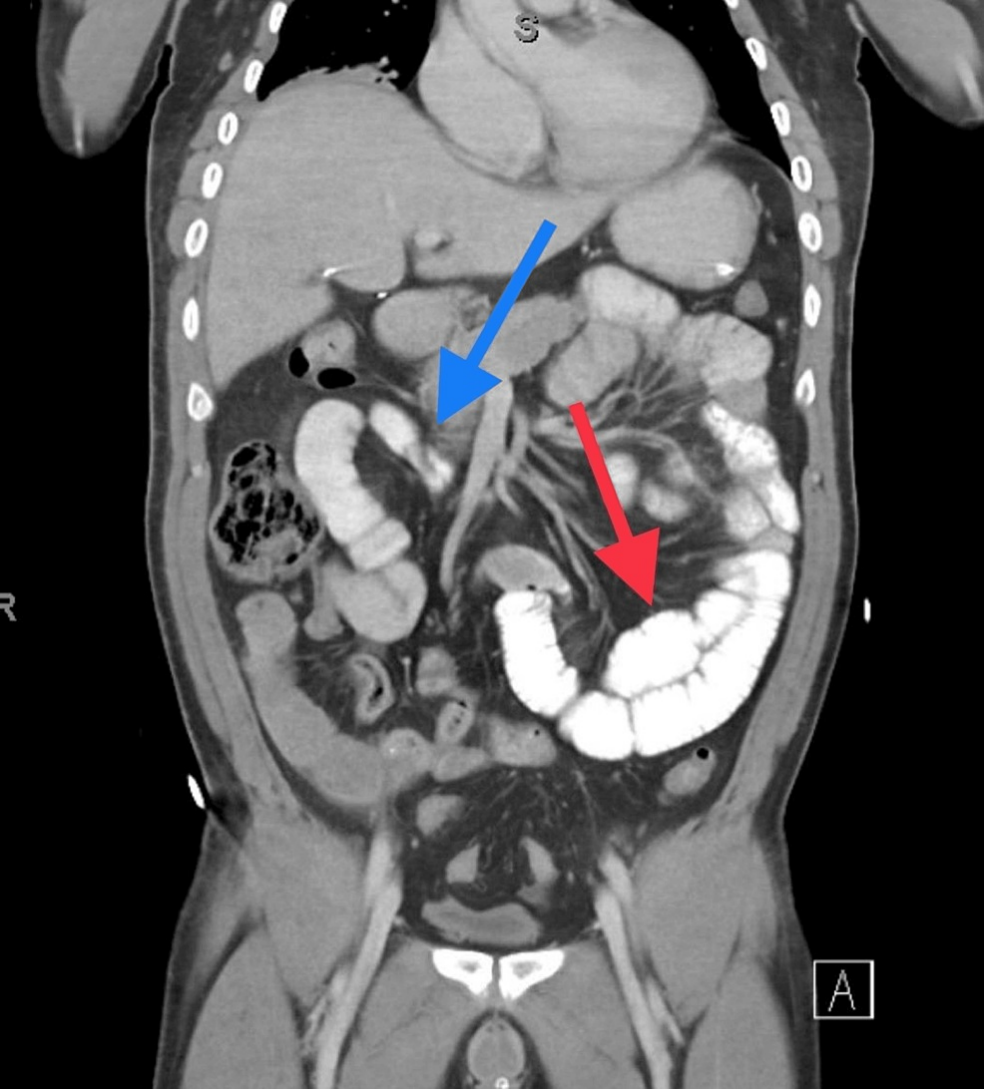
A CT scan abdomen pelvis showing small bowel obstruction with contrast within the dilated loops of the small bowel (red arrow) but no contrast noted within the distal decompressed small bowel loops (blue arrow).
